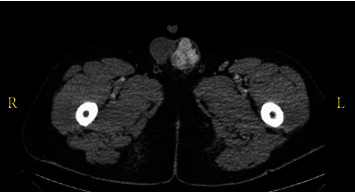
CT scan of the abdomen and pelvis revealed a large lobulated mass in the left scrotum.
